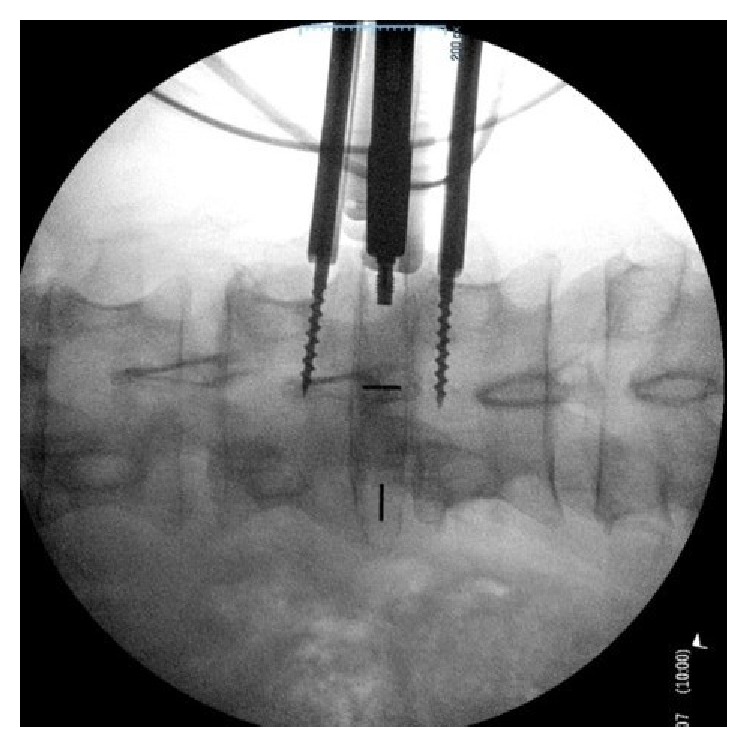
Intraoperative radiograph of PEEK Cage Insertion using RAVINE.
